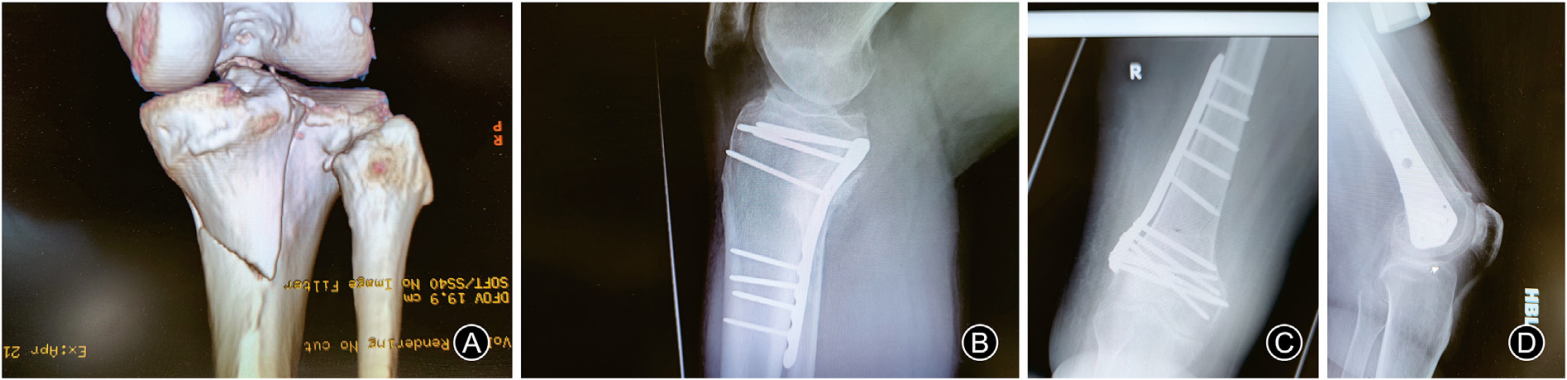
Preoperative (A) and postoperative (B) images of a posteromedial tibial fracture operated with posteromedial plating; preoperative (C) and postoperative (D) X-ray for another case of distal femur fractures.
